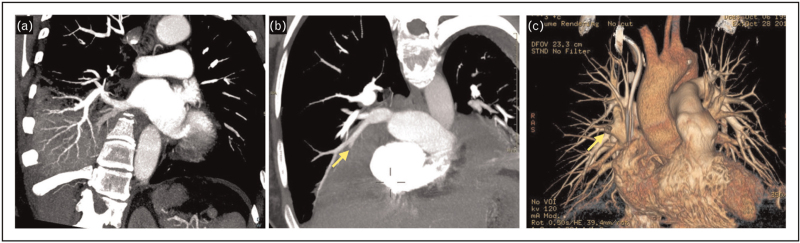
Computed tomography pulmonary angiography for detection of invasive fungal disease. (a) Negative vessel occlusion sign as patent contrast-enhanced vessels are visualized in the right lower lung consolidation of a neutropenic patient with *Pseudomonas aeruginosa* pneumonia; (b) positive vessel occlusion sign (arrow) in the right lung of a neutropenic patient with positive serum galactomannan (probable aspergillosis); (c) volumetric projection of the pulmonary vessel tree demonstrating obstructed vessel (arrow).
